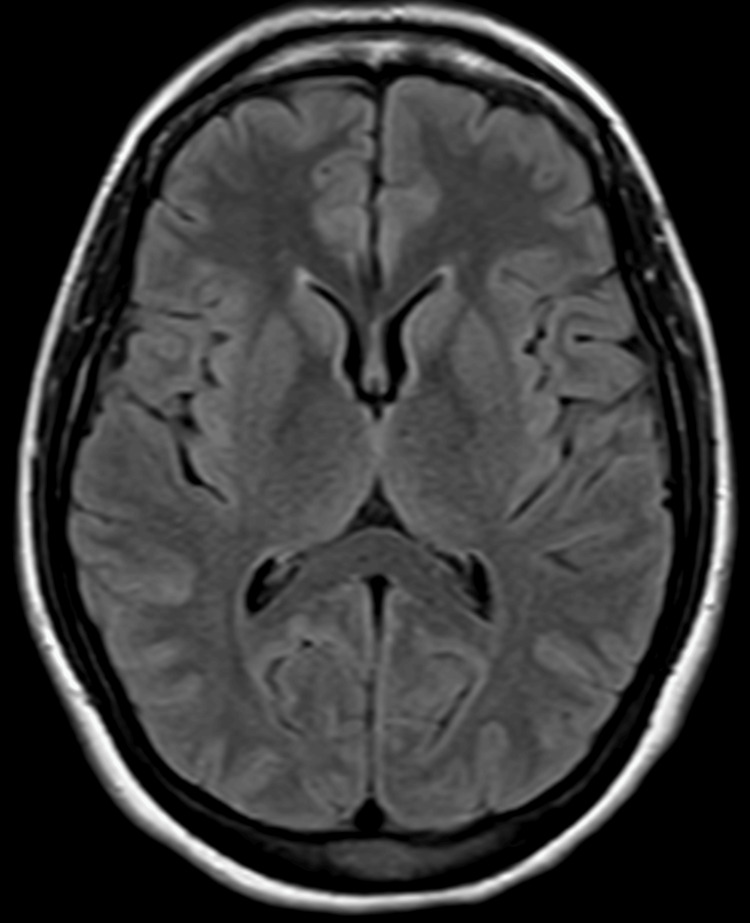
Axial brain MRI, FLAIR sequence, reported as within normal limits FLAIR: Fluid-Attenuated Inversion Recovery.
